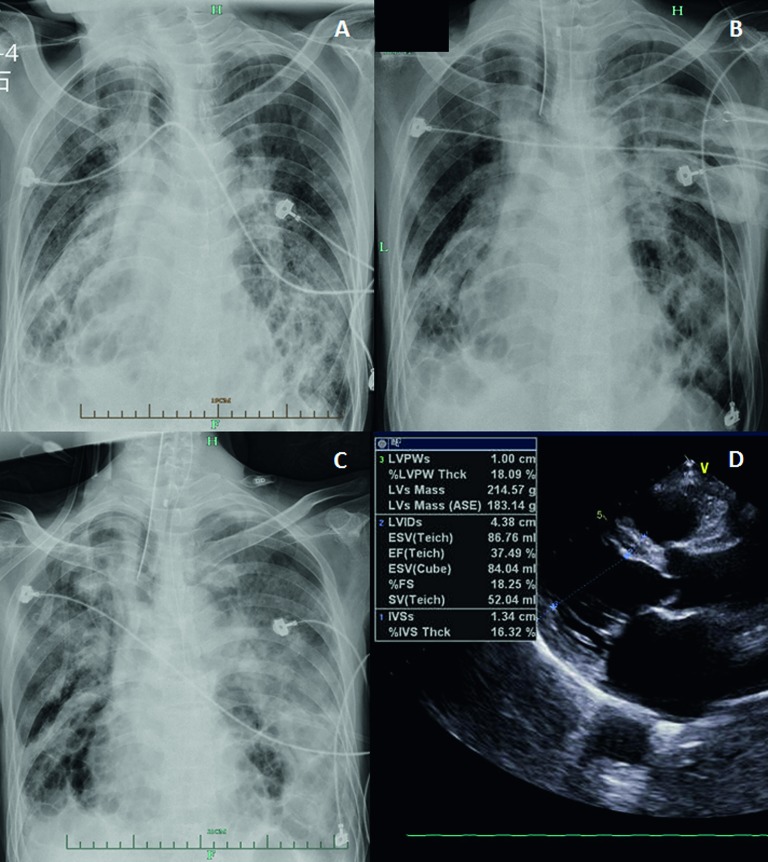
Radiographic and ultrasound findings in case 2. (A) Chest radiograph at the time of admission showed bilateral pulmonary infiltration. (B) Chest radiograph on day 2 compared with that at admission showed progression of the bilateral pulmonary infiltration and consolidation. (C) Chest radiograph on day 4 showed progression of bilateral pulmonary infiltration and consolidation compared with day 2. (D) Echocardiogram at the time of admission showed cardiac failure (LVEF 37.5%). LVEF, left ventricular ejection fraction.
